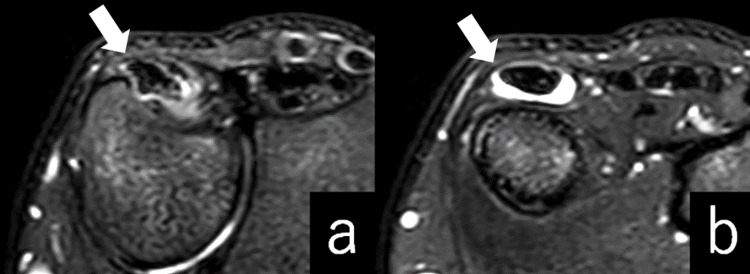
Preoperative T2-weighted fat-suppressed MRI showing the level of ECU groove. ECU tendon is deformed (a) and surrounded by reactive fluid or proliferated synovium (b). ECU: extensor carpi ulnaris.
